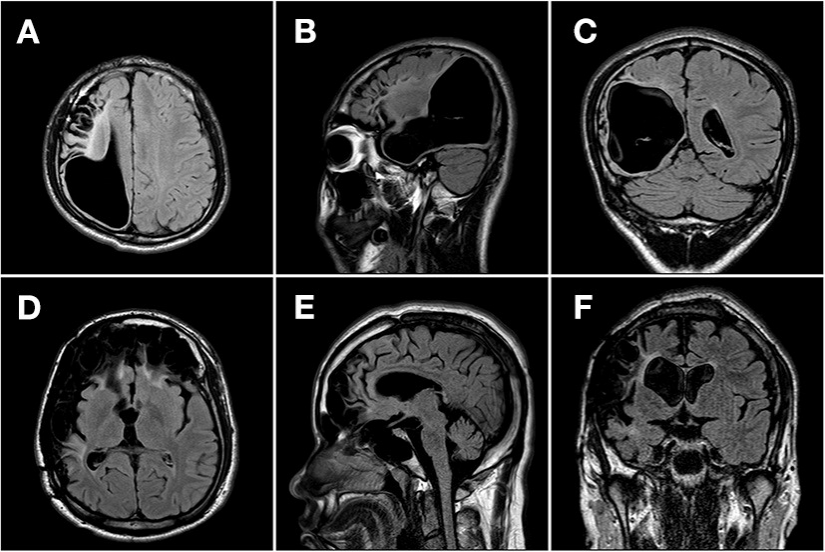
Representative MR images of patients with pharmacoresistant epilepsy secondary to encephalomalacia. Representative MR images (T2-weighted fluid-attenuated inversion recovery image) of two patients with pharmacoresistant epilepsy secondary to encephalomalacia in the axial (A, D), sagittal (B, E), and coronal (C, F) planes. (A–C) A 17-year-old boy with unilateral encephalomalacia on MRI due to intracranial hematoma. The encephalomalacia was observed in the right frontal, parietal, and temporal lobes. The patient got a reduction of 80% in seizure frequency after 1 year following the VNS therapy. (D–F) A 24-year-old man with bilateral encephalomalacia on MRI due to head trauma. The encephalomalacia was observed in the right temporal and parietal lobes, as well as in bilateral frontal lobes. The patient got no reduction in seizure frequency during a 3-year follow-up after the VNS therapy.
